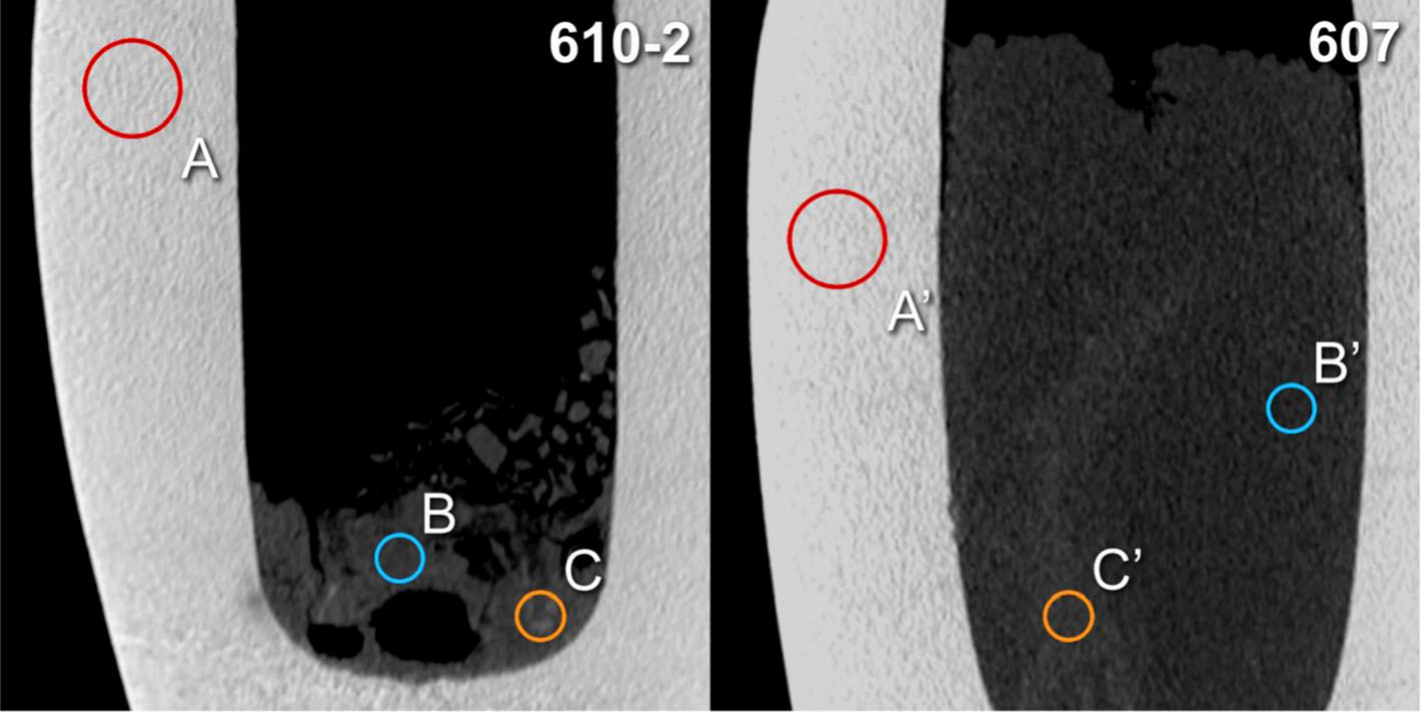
CT-based density measurements. Placement of regions of interest A and A’ (calcite mineral), B and B′ (surrounding material) and C and C′ (structures of higher radiodensity) for two canopic jars (inventory numbers 610-2 and 607) on sagittal multiplanar reconstructions (slab thickness 1.5 mm)
